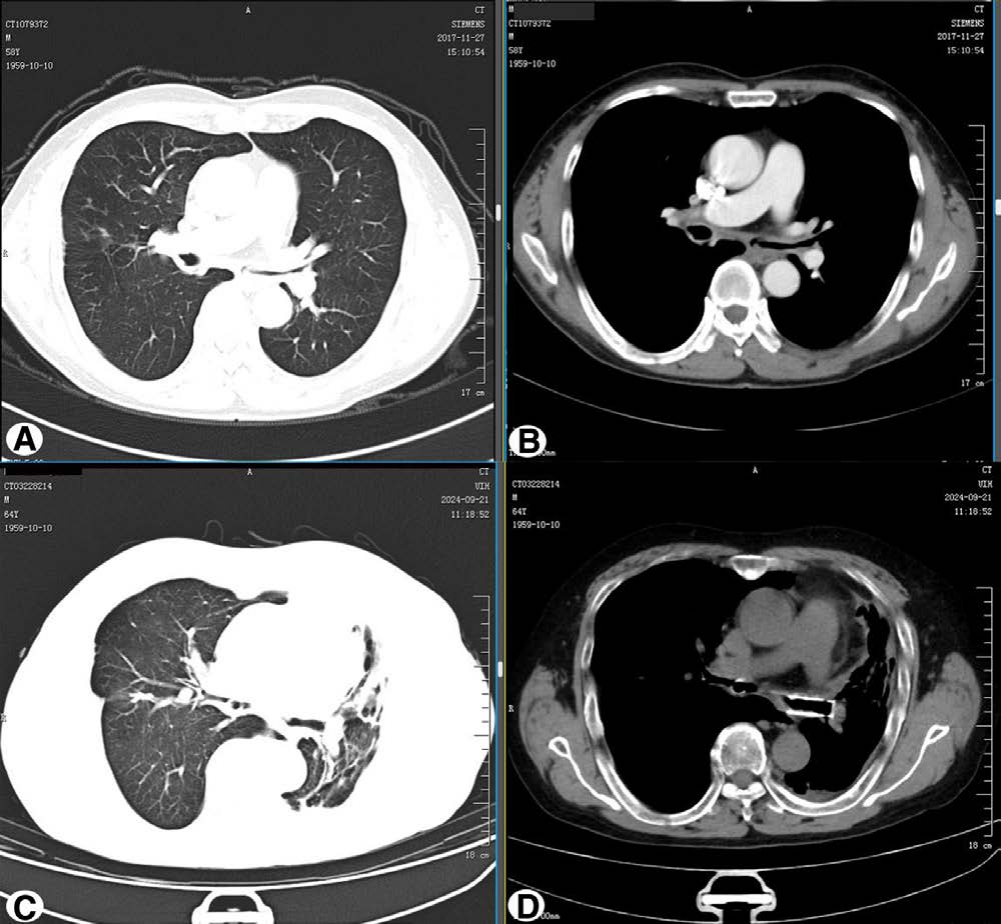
(A and B) Chest CT showed thickening of the tracheal and bronchial walls with narrowing of the lumen. (C and D) Chest CT showed atelectasis and bronchiectasis of the left lung, with chronic infection. CT = computed tomography.
